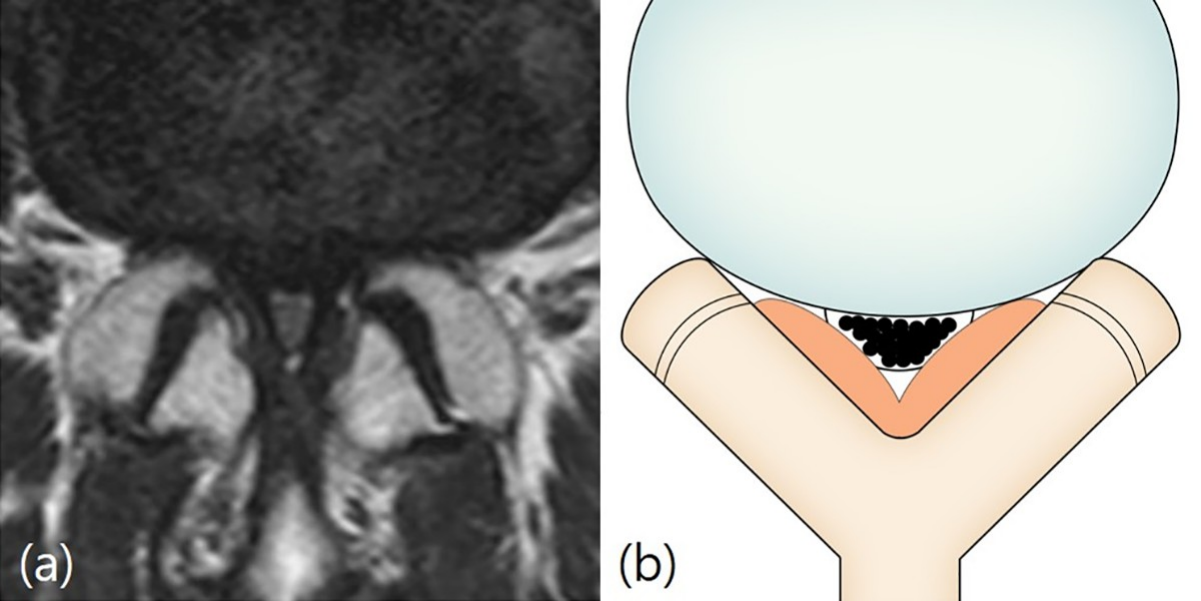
Lee classification. Grade 3 (severe stenosis). Fig 6A and 6B: A 69-year-old woman with pain in both lower extremities. T2-weighted axial magnetic resonance image at L4/5 disc level shows severe obliteration of the anterior cerebrospinal fluid space, marked compression of the dural sac, and the entire cauda equina appearing as one bundle.
